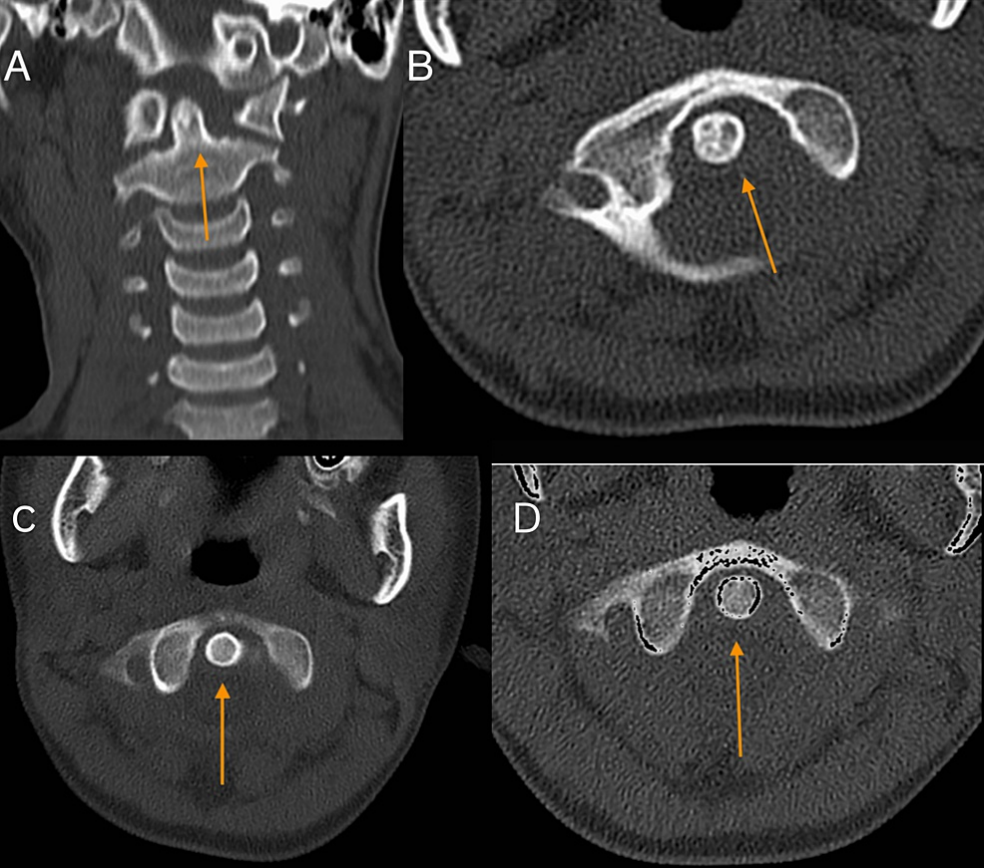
(A) Coronal and (B) axial cut section of the CT scan of the cervical spine eight weeks post-injury. It was noted that there was an improvement in the C1-C2 rotatory dislocation (arrow). (C) Axial cut section of the repeated CT scan two weeks post-closed reduction showed that the atlantoaxial rotatory dislocation improved (arrow). (D) The axial cut of the CT scan six weeks post-closed reduction showed a successful reduction (arrow). CT: computed tomography
